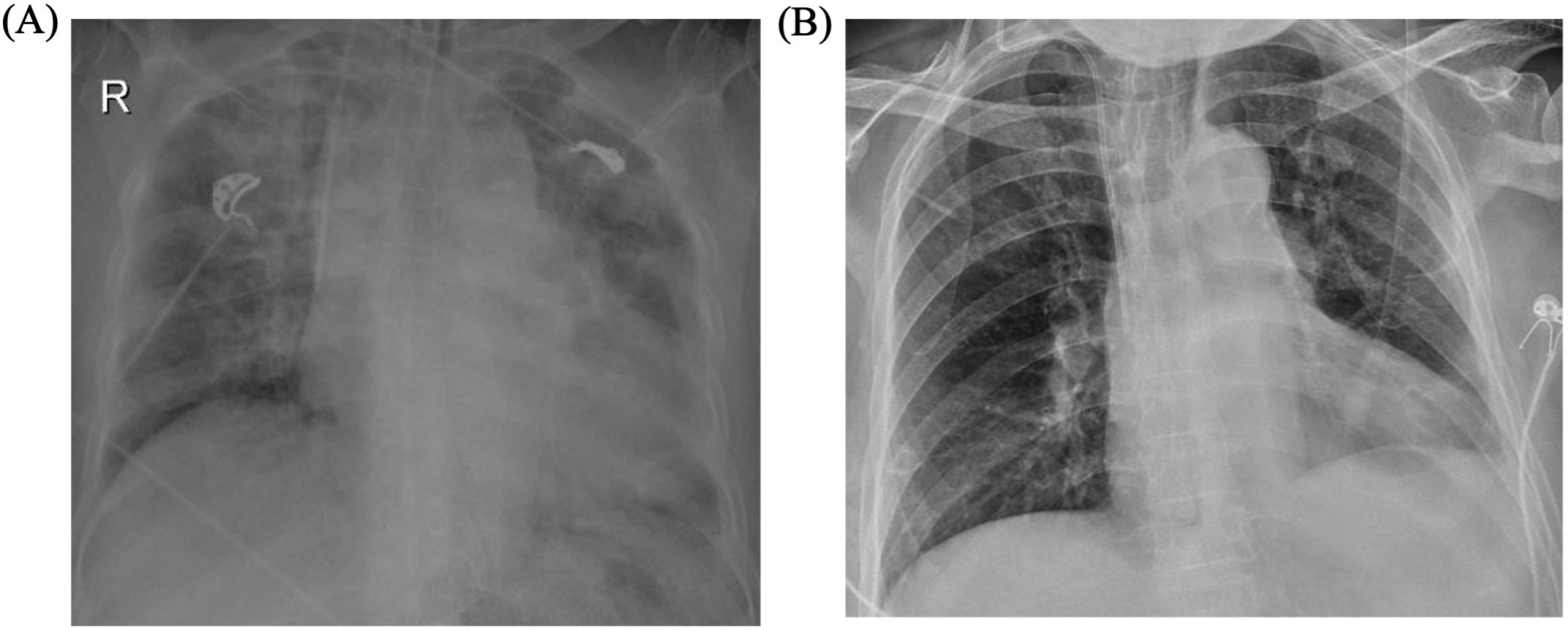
CXR on admission (A) and before discharge (B) CXR, chest x-ray.
